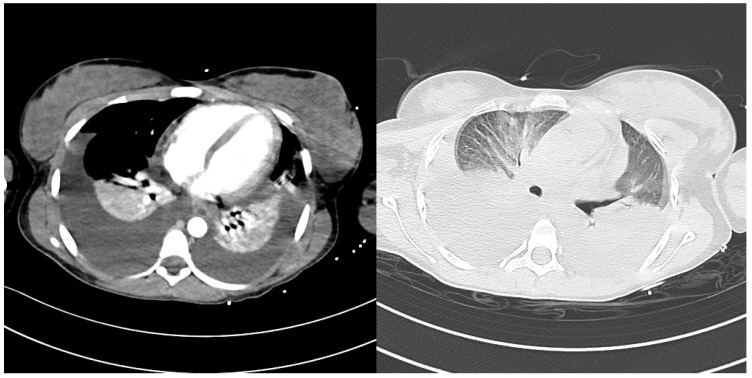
CT image of bilateral pleural effusion.
